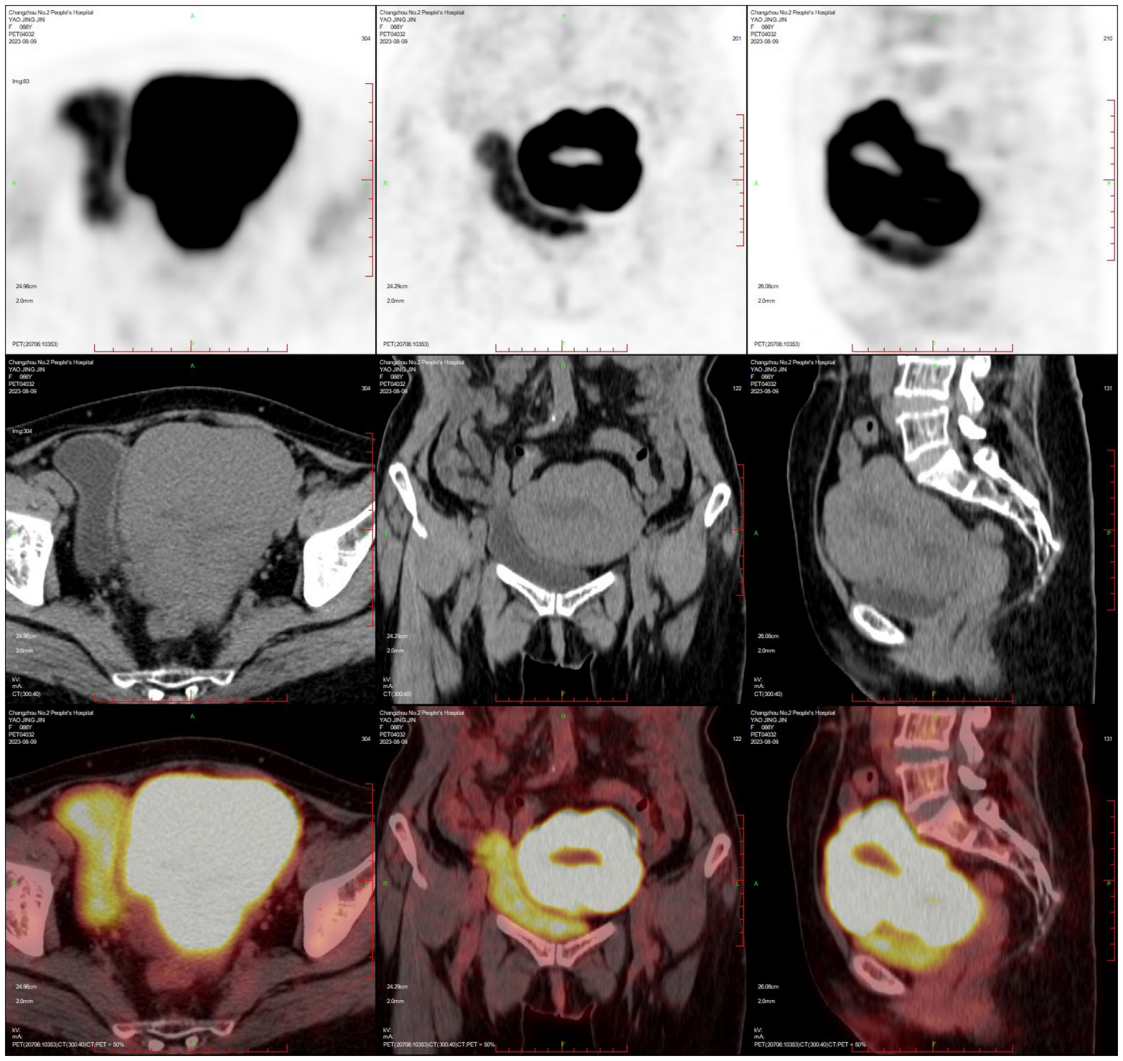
Uterine lymphoma PET-CT.
